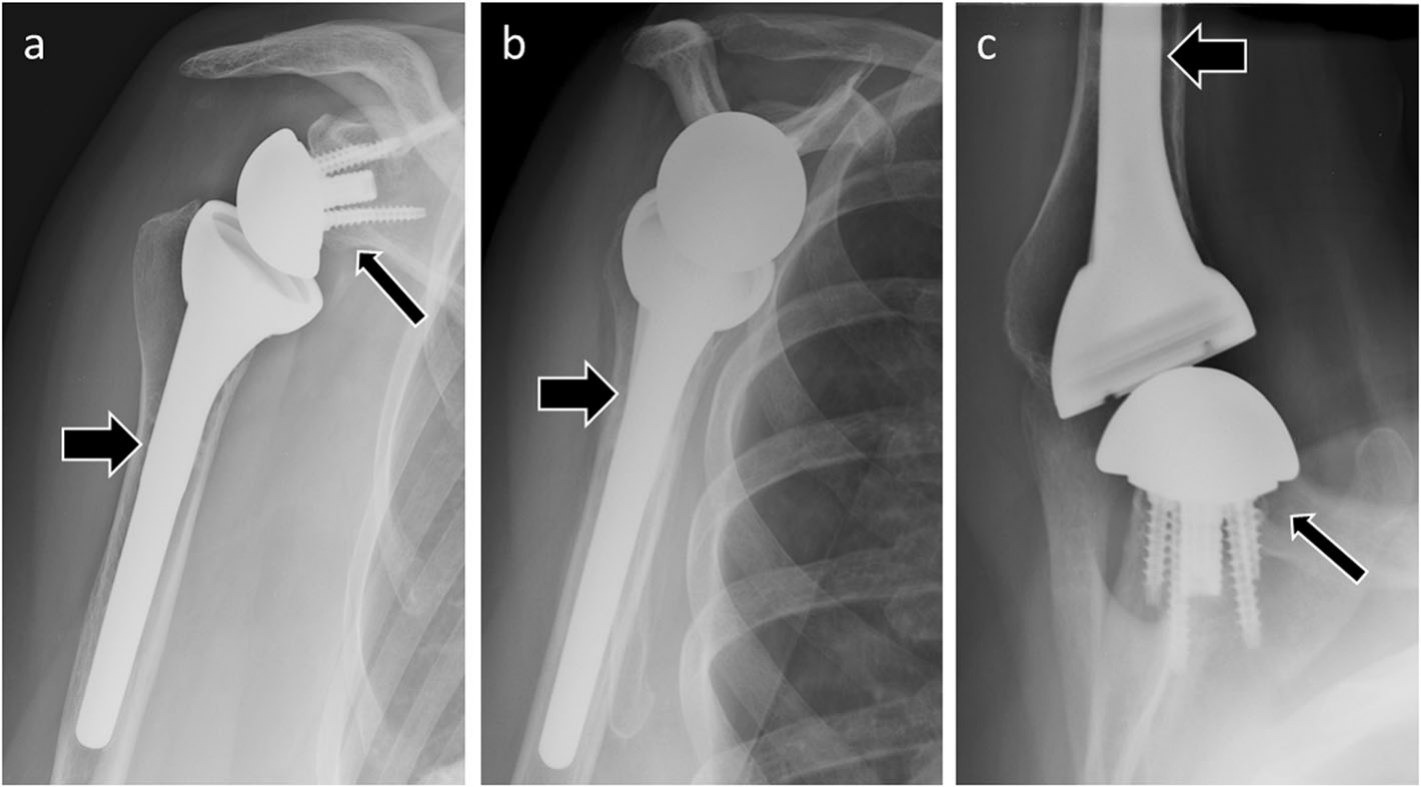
Anteroposterior (a), scapular Y (b), and axillary (c) radiographs of normal findings in a patient with reverse shoulder arthroplasty. The humeral prosthetic stem (thick arrows) is centered within the humeral shaft. The glenosphere (thin arrows) is placed flush with the native glenoid (or slightly lowered) and the humeral component is centered on the glenosphere
